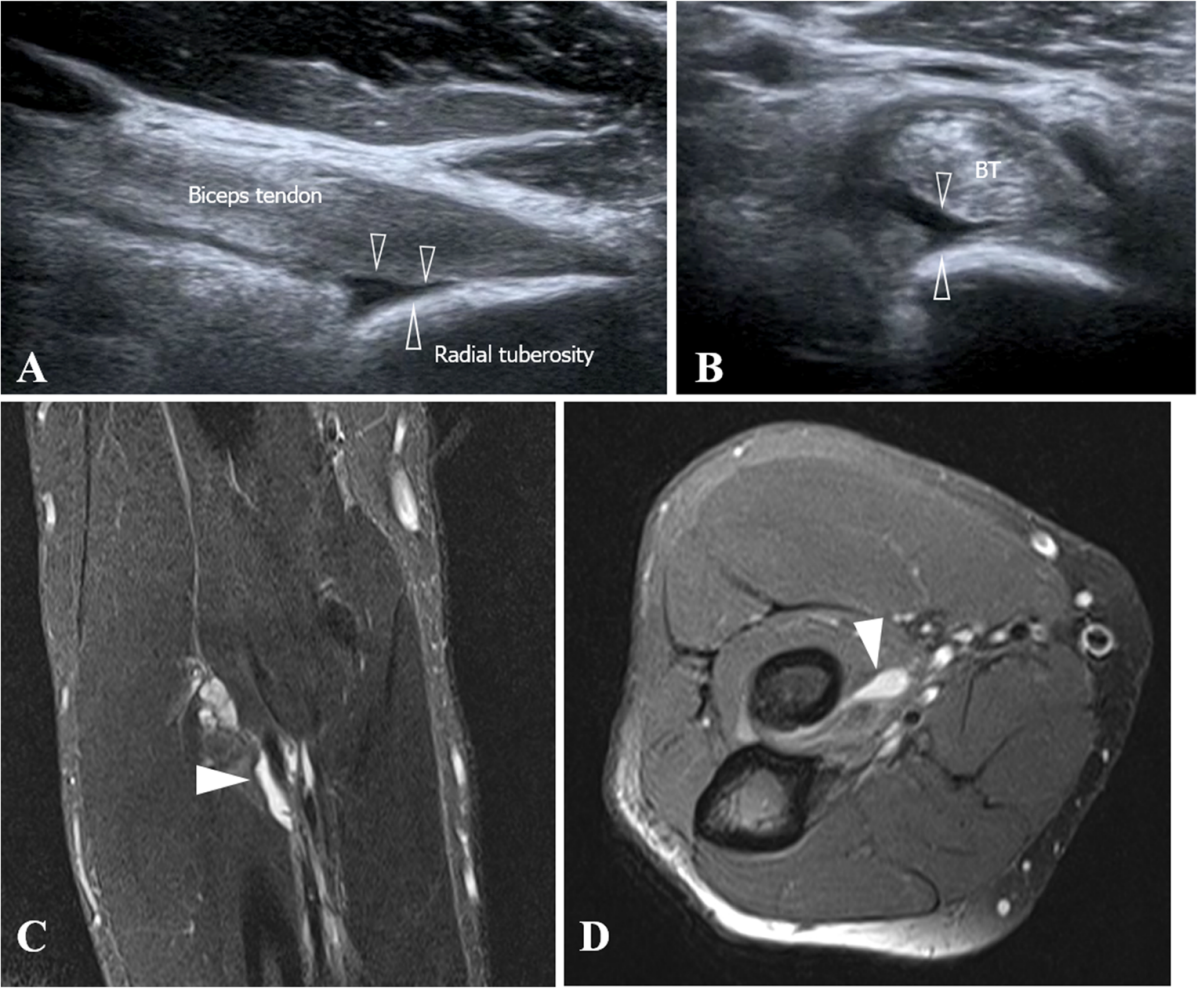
Ultrasound and MRI views of a type 2A injury (low-grade partial tear at the attachment). a In the long-axis view, a minor partial detachment (*arrowheads*) affecting less than 50% of the DBBT (biceps tendon), is observed just adjacent to the radial tuberosity. The minor partial detachment can be differentiated from other pathological processes as it is presented as a mild focal thinning of the DBBT just adjacent to the radial tuberosity. The tendon defect is then filled with anechoic fluid. b Short-axis view of a. The cross-section view also demonstrates the minor partial detachment (*arrowheads*) and a better view of a mild peritendinous effusion that could also be interpreted as a bicipitoradial bursitis secondary to synovial irritation by bleeding after the tear. c, d Elbow MRI, coronal and axial PD with FS-weighted sequences (respectively). DBBT thickening and contour alteration can be observed at the attachment site. At that point an intratendinous hyperintense signal (*arrow*), similar to that of fluid, shows a tendon defect affecting less than 50% of the DBBT and therefore compatible with a low-grade partial detachment type 2A
